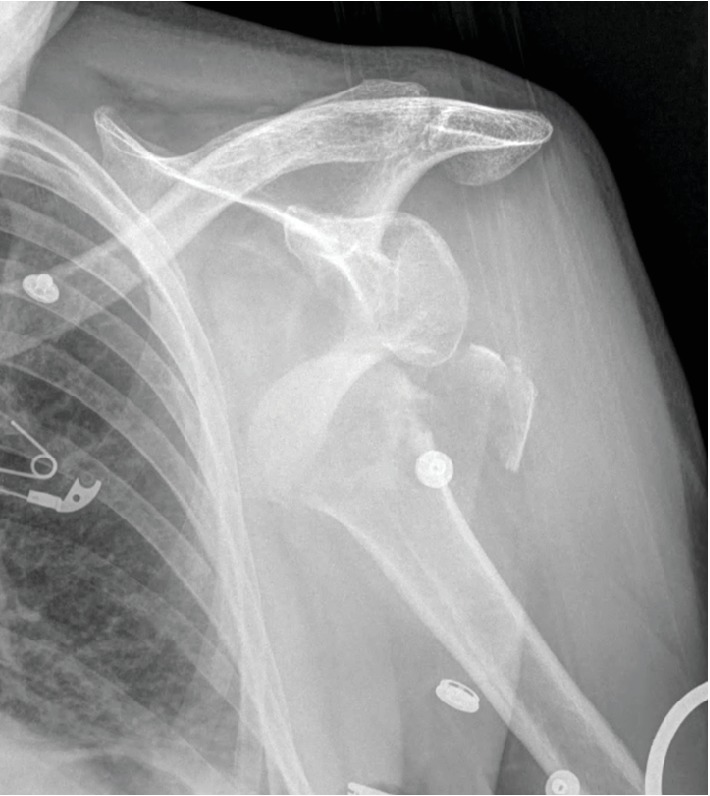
Four-part fracture of the left proximal humerus. A plain radiograph of the left shoulder taken in the anteroposterior (AP) direction at presentation showing a displaced, four-part fracture of the proximal humerus with involvement of the surgical neck and greater and lesser tuberosities.
